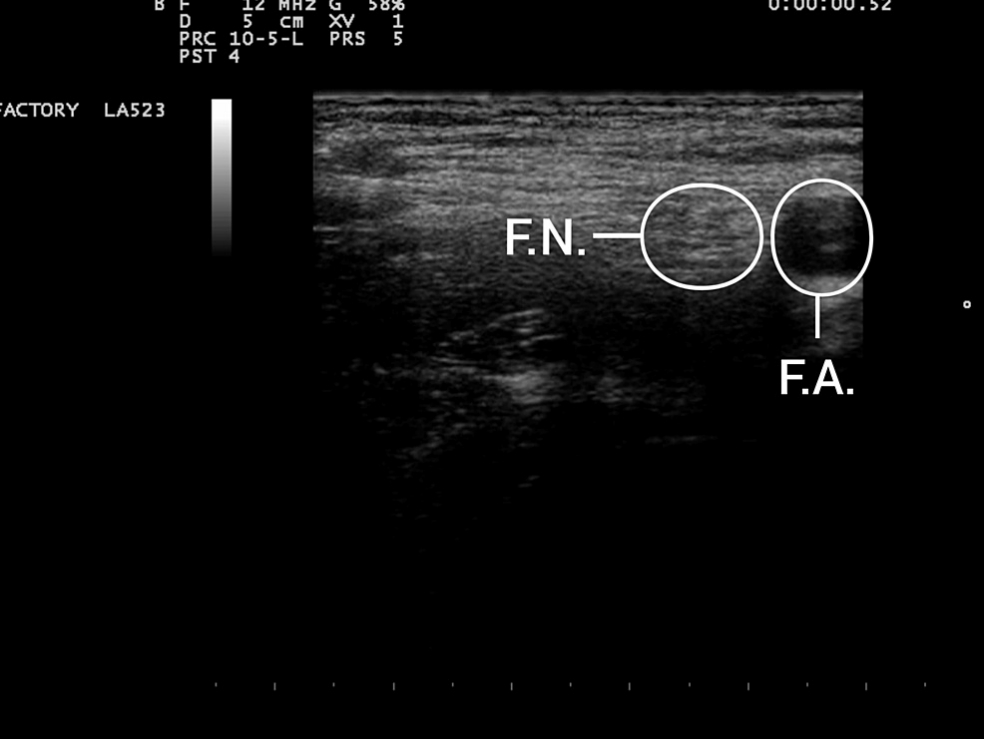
Sonographic picture of FN. FN, femoral nerve; FA, femoral artery
